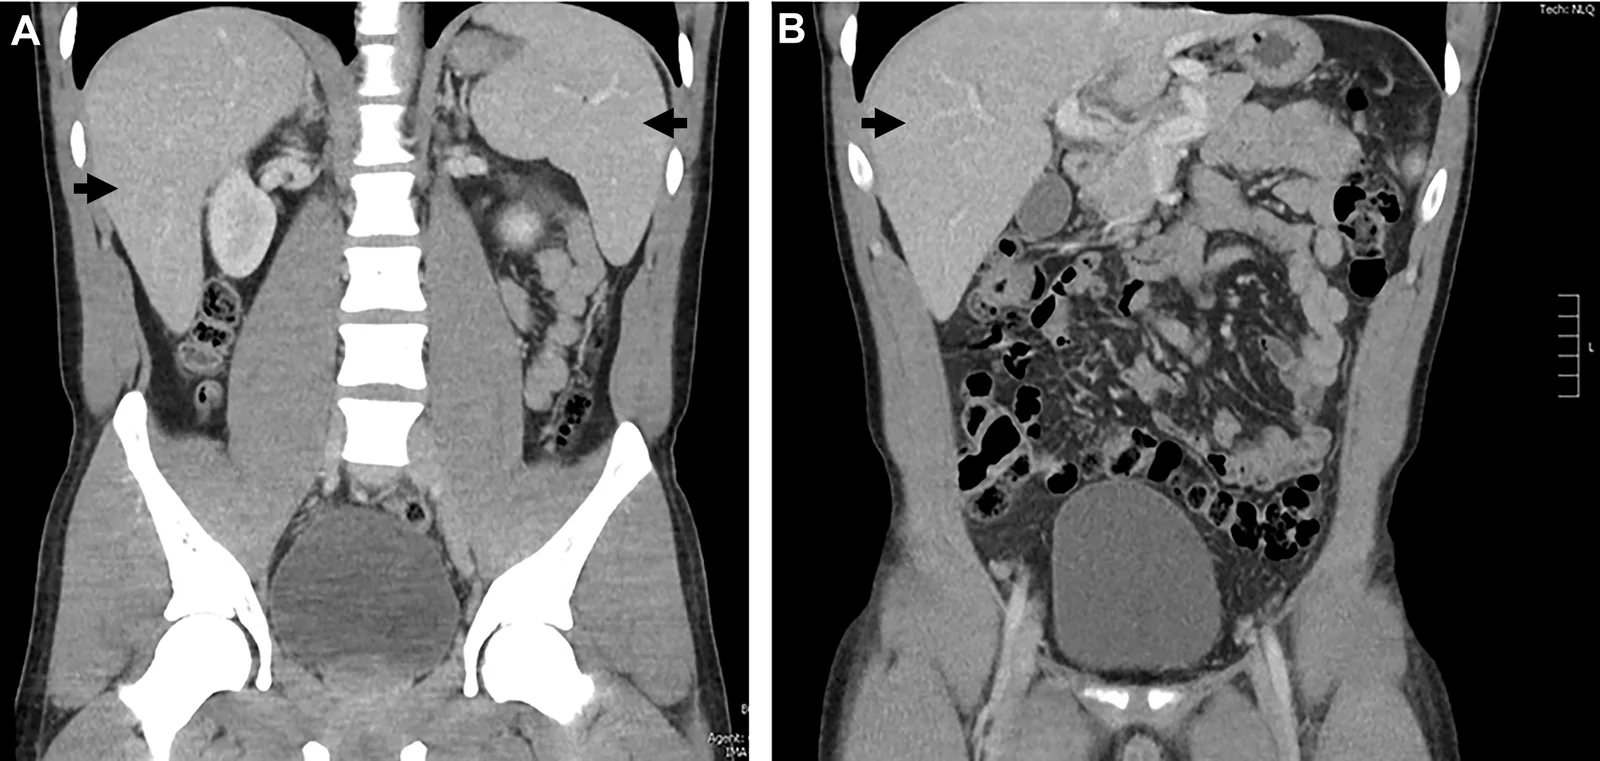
CT of the abdomen and pelvis with intravenous contrast No abnormality was seen, including no calcifications in the liver or spleen. Two cuts are presented ((A) anterior; (B) posterior). Rightward arrows indicate the liver; leftward arrow indicates the spleen. CT: computed tomography
